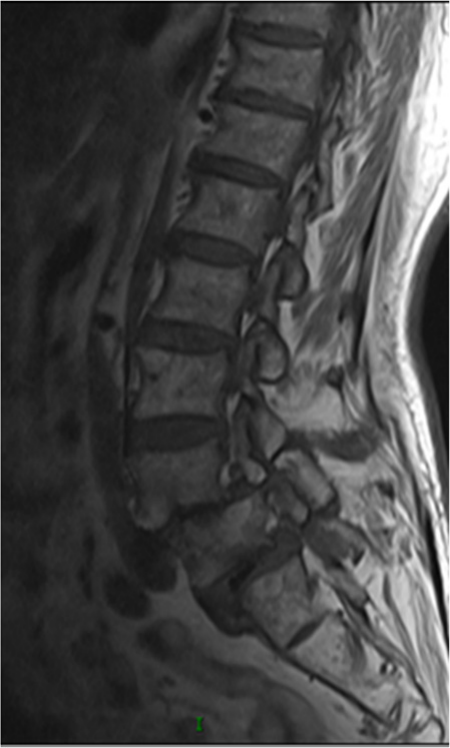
Magnetic resonance image shows II-degree spondylolisthesis at L4 and L5, and the corresponding segment of the nerve compression
